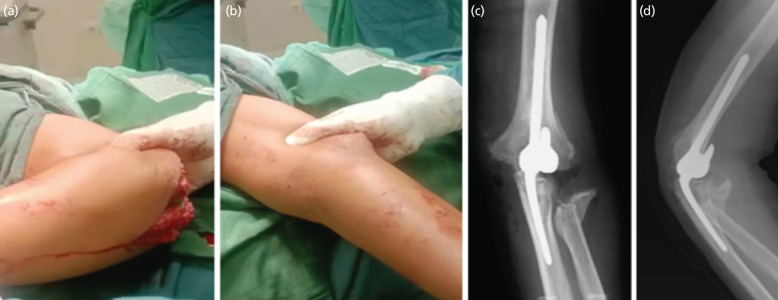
(a, b) Intra-operative image showing passive ROM of 0/100. (c) Post-operative radiograph in AP, and (d) lateral view.
